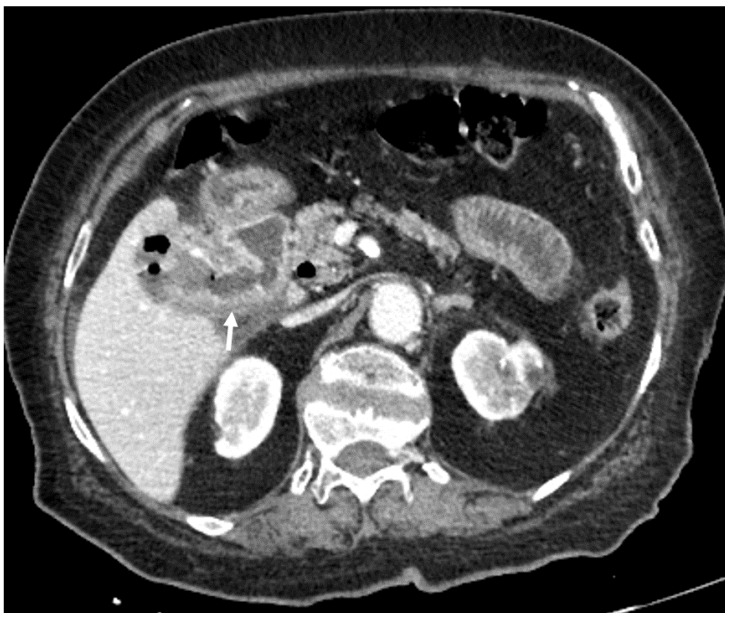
87-year-old female. Abdominal CT scan, axial plane, portovenous phase. Collapsed gallbladder, with thickened walls, pericholecystitis and air in the pericholecystic space. Also, a connecting path between the gallbladder and duodenum is visible (arrow)—cholecystoduodenal fistula.
